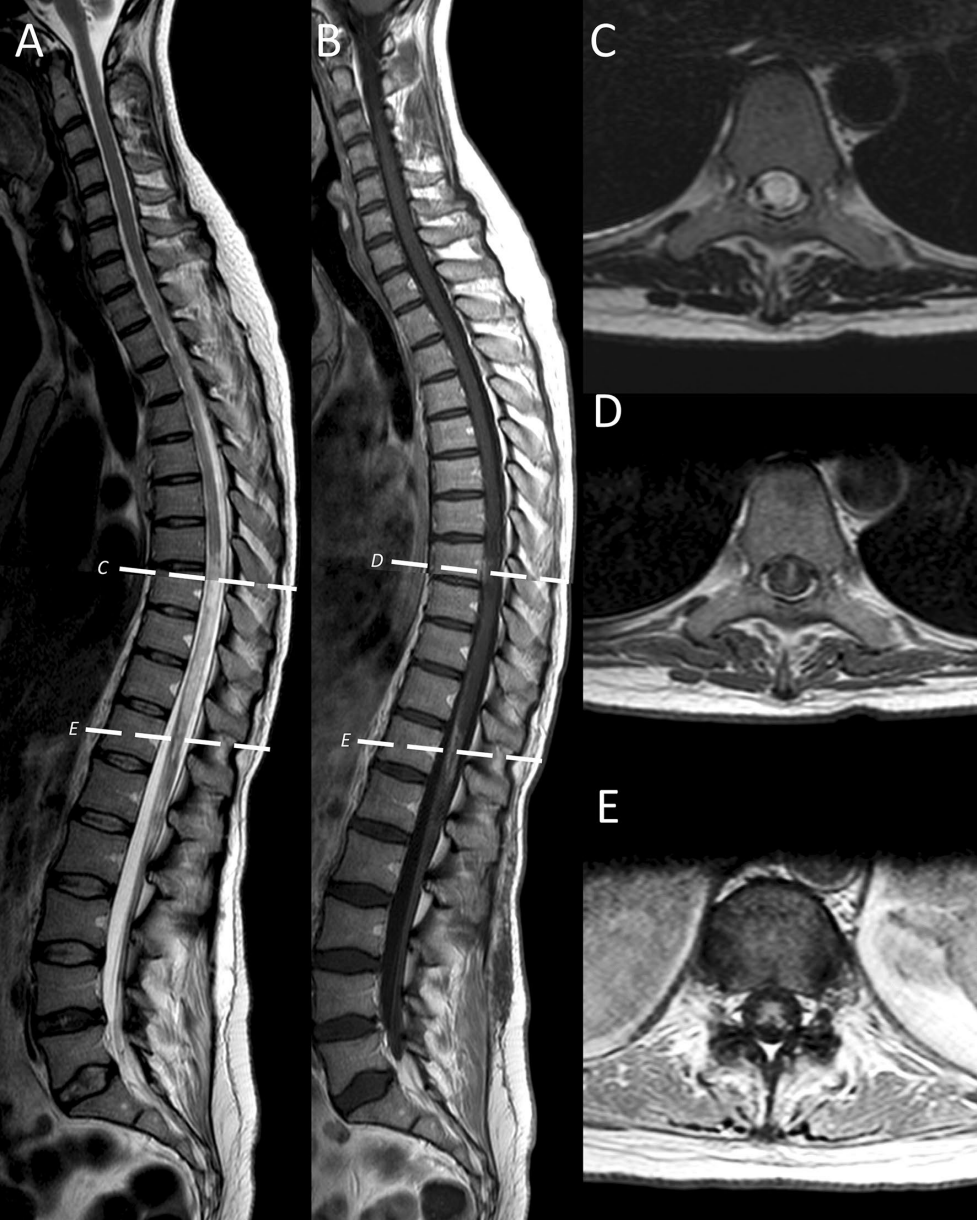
Two months after subacute onset follow-up spinal MRI (no steroid therapy) shows severe chronic LETM with still some active inflammation at the same sites. From left to right: compared to the images at subacute onset (Fig. 2; with steroids) sagittal spinal MRI (1.5T, T2w) revealed much stronger and more extensive longitudinal T2w signal intensities (A) throughout the thoracic myelon from Th7 to the conus, and in (B) stronger enhancement in the conus and at TH8 in the T1w images with contrast. The transverse slices on the right show the corresponding slices at the level of TH8 and the conus indicated by the white hatched lines in the sagittal images. C Transverse T2w images; D, E transverse T1w images with contrast at the levels indicated in the sagittal slices
